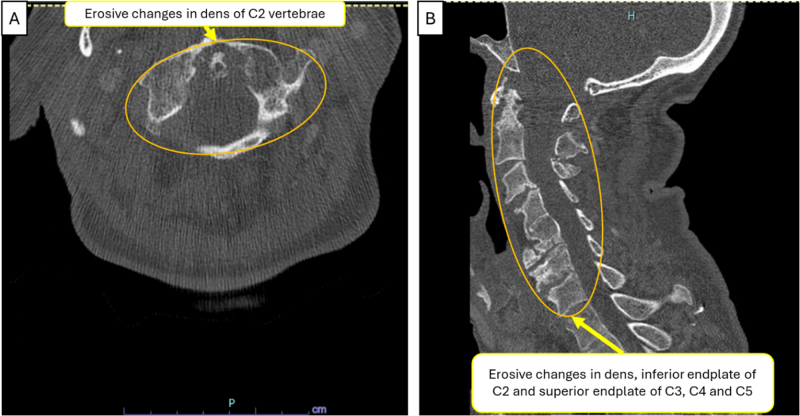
CT cervical spine [axial (A) and sagittal (B)] view showing erosive changes in dens and inferior endplate of C2, extending to superior endplate of C3, C4, and C5, suggestive of inflammatory arthritis
